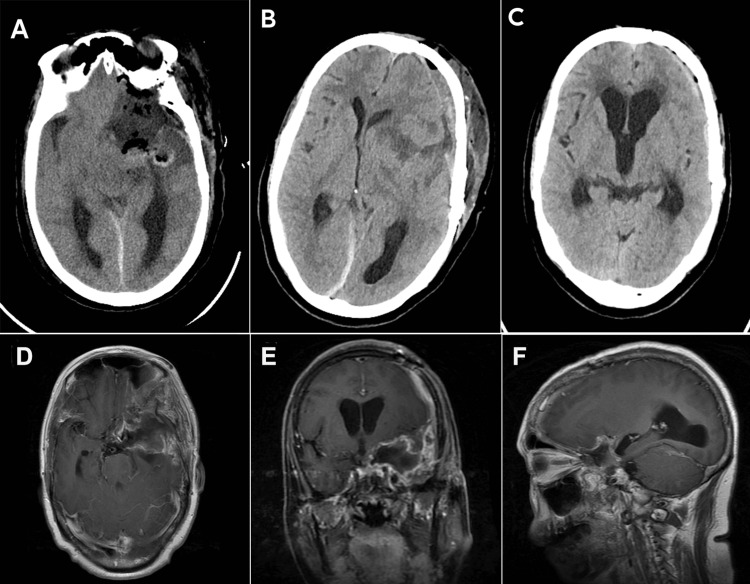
Postoperative images A: postoperative CT at three days; B: postoperative CT at 10 days; C: postoperative CT at one month; D-F: postoperative enhanced MRI at one month
